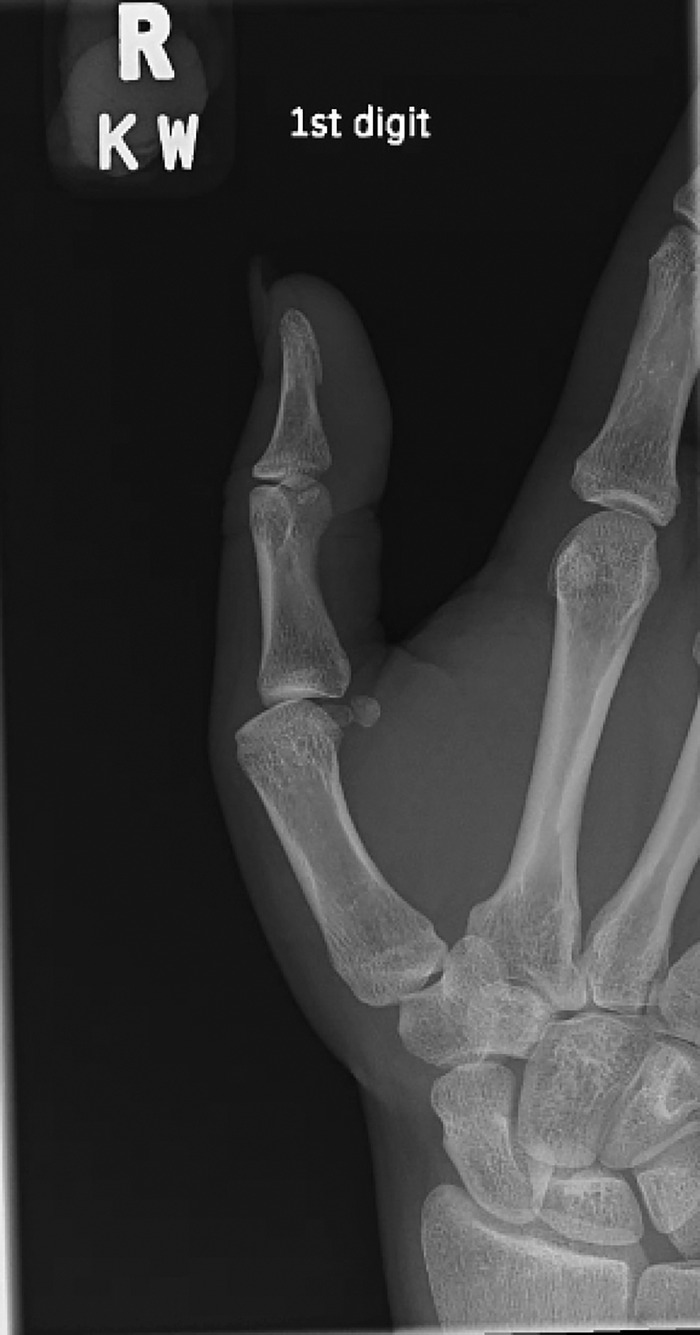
Preoperative radiograph of the right thumb shows subluxation of the metacarpophalangeal joint.
